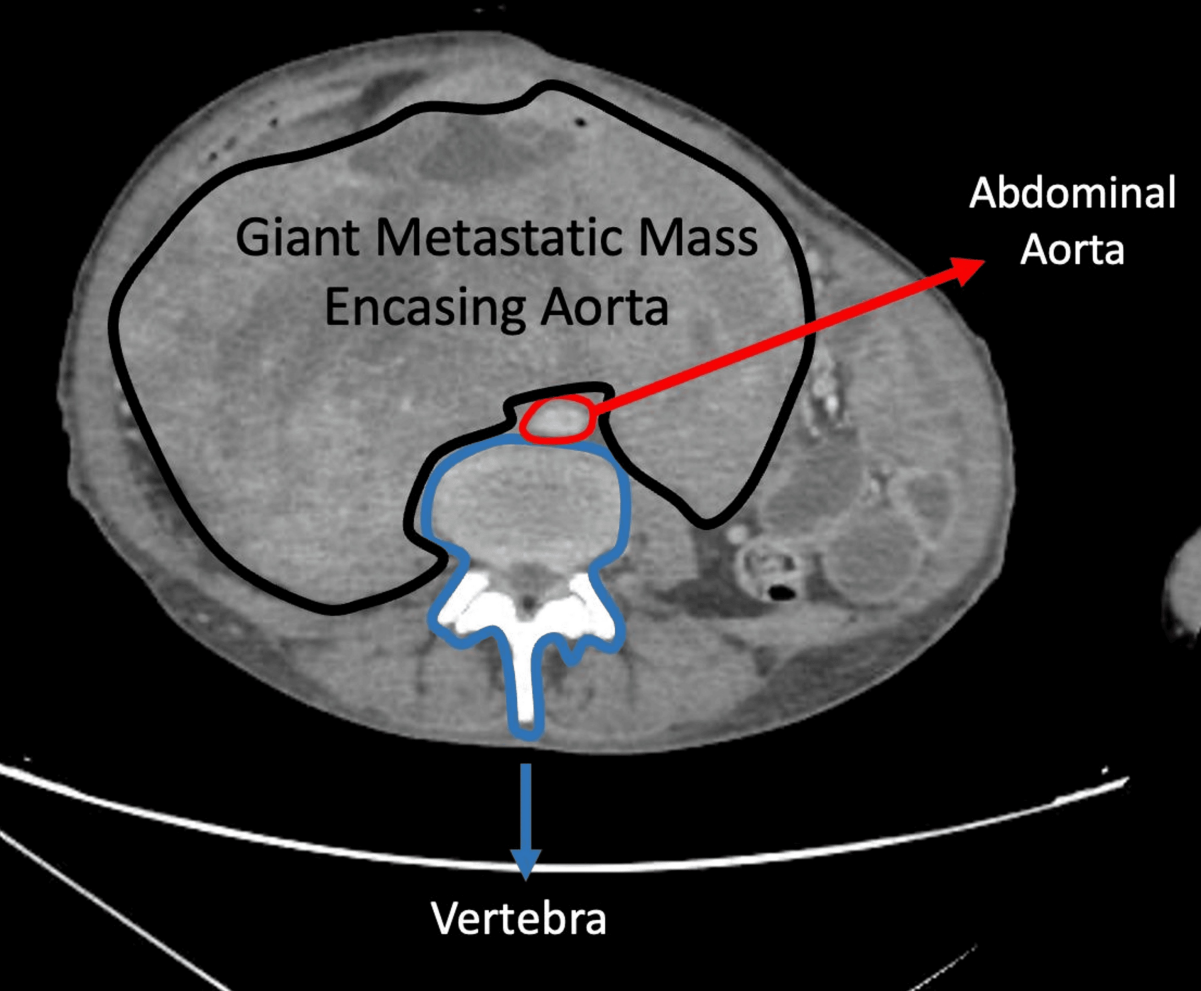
Contrast-enhanced axial CT image demonstrating a large retroperitoneal mass Post-contrast axial image showing a large mass encasing the aorta, extending into the paravertebral region, filling the right hemiabdomen, and displacing the small-bowel loops to the left
